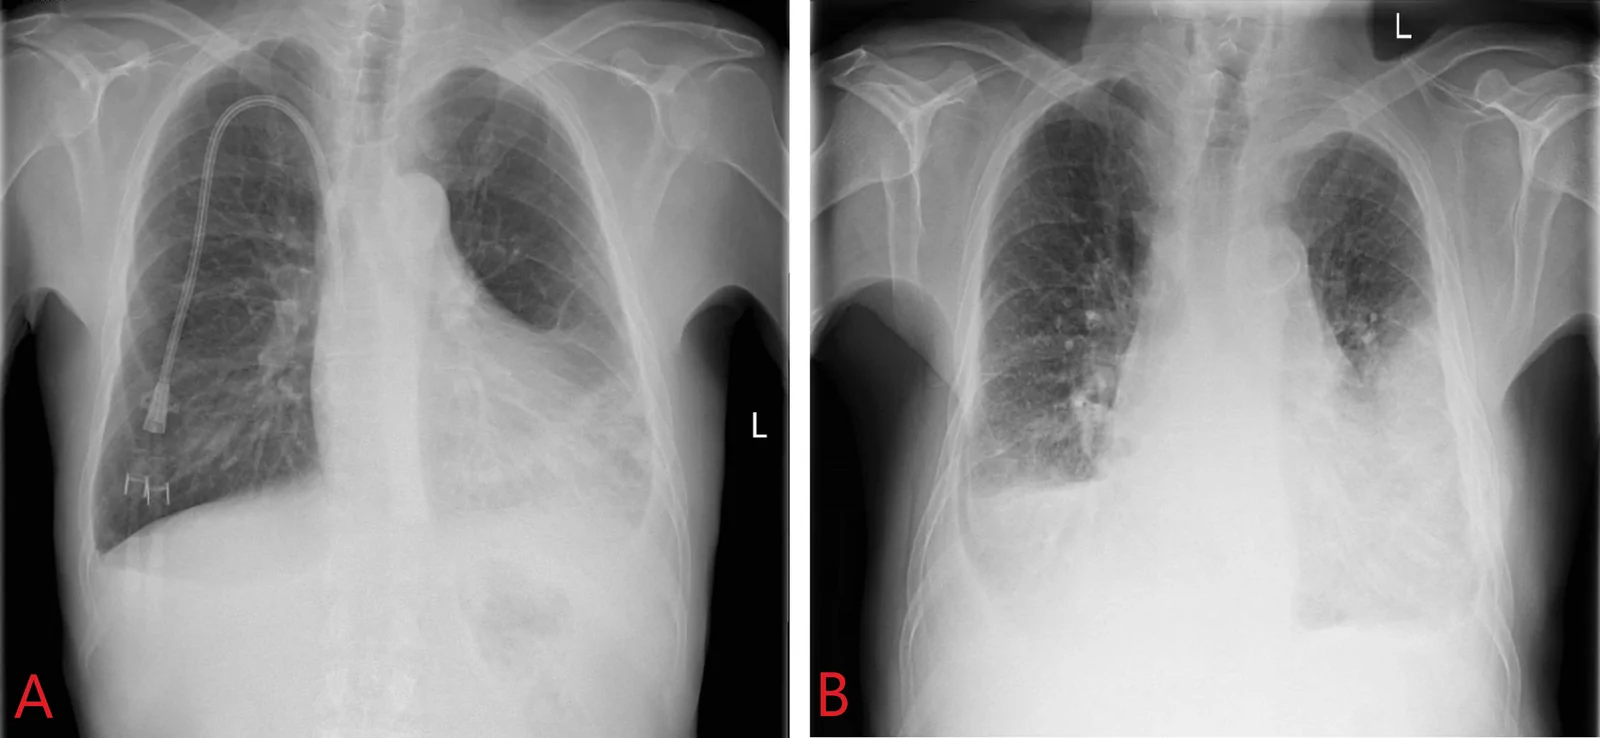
Chest radiographs. (A) January 2019 (A) and (B) July 2025, showing persistent left-sided mid-to-lower zone opacification, which is representative of the organised pleural haematoma.
